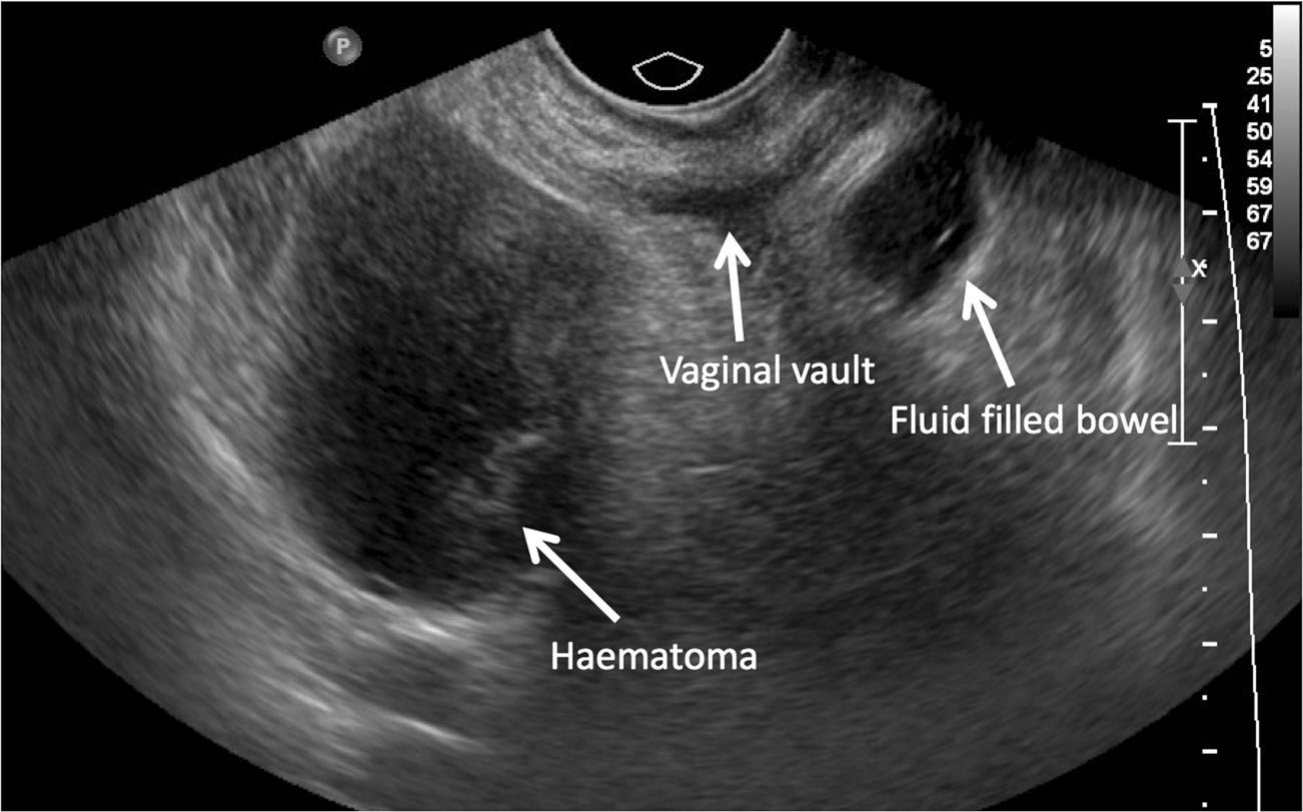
Predrainage transvaginal ultrasound scan of haematoma = 5 cm (ultrasound depth 7.1 cm, making haematoma more magnified)
